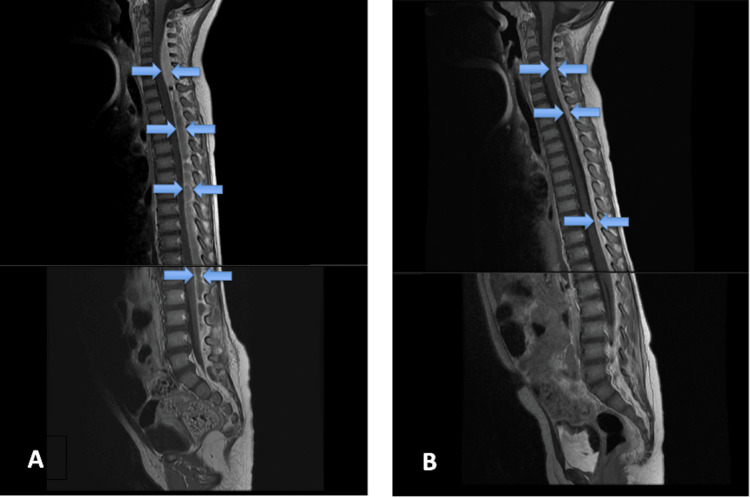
Follow-up contrast-enhanced, sagittal T1-weighted MRI views. (A) On day 10, there is a reduction in epidural collections at the thoracic, lumbar, and sacral levels, with a small residual collection at the cervical level. The dura mater appears thickened with evident contrast enhancement. (B) On day 15, there is further reduction of the cervical epidural pus, and the cord is nearly fully decompressed.
